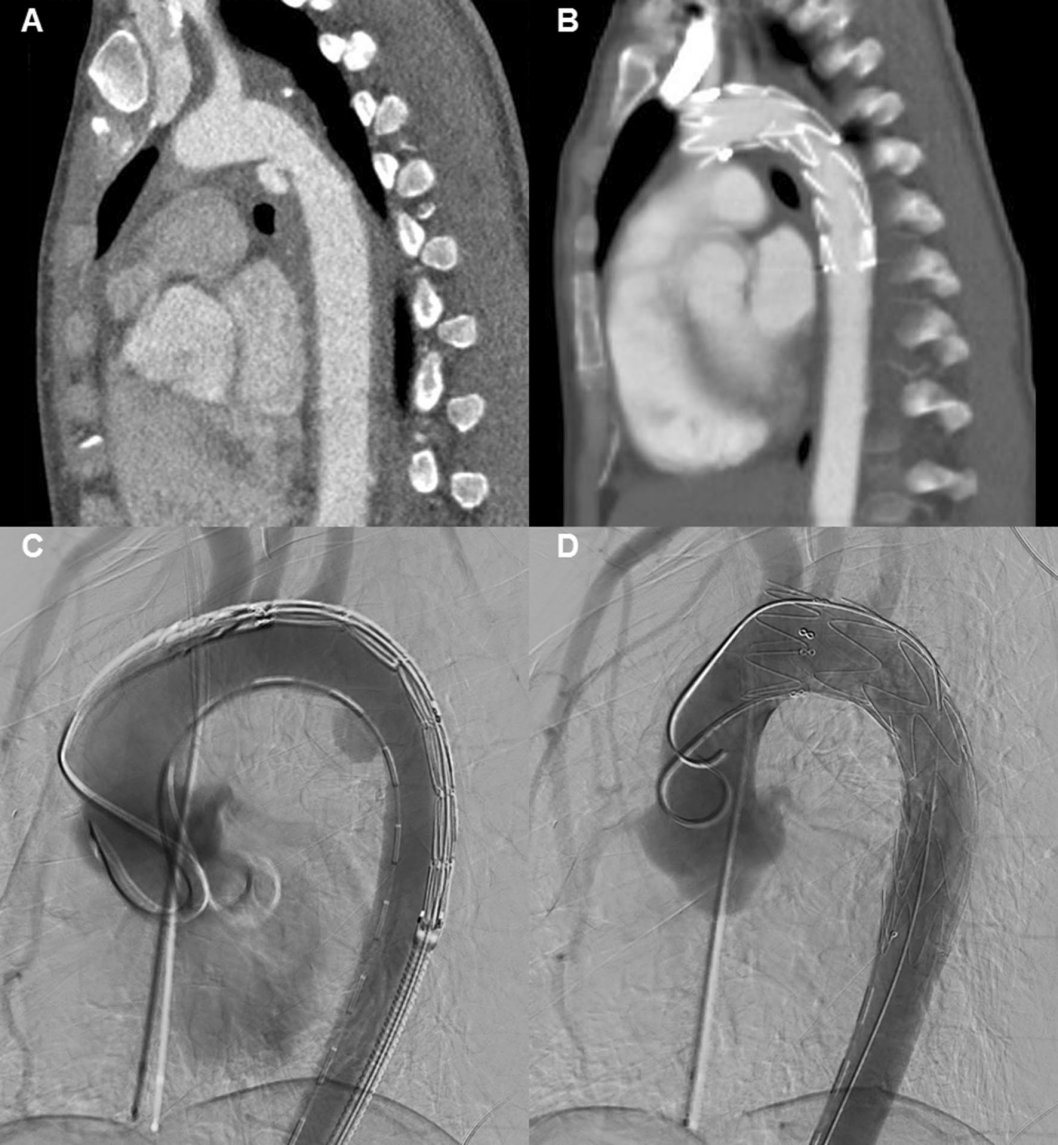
a Pre-operative CTA scan of the thoracic aorta, displaying a grade III injury (pseudoaneurysm, without extravasation) located near the isthmus. b Post-operative CTA scan, displaying pseudoaneurysm coverage by TEVAR. c Procedural angiography displaying the aortic pseudoaneurysm before stent graft deployment. d Procedural angiography after proximal bare stent graft deployment, covering the pseudoaneurysm and left subclavian artery (visible due to retrograde flow)
